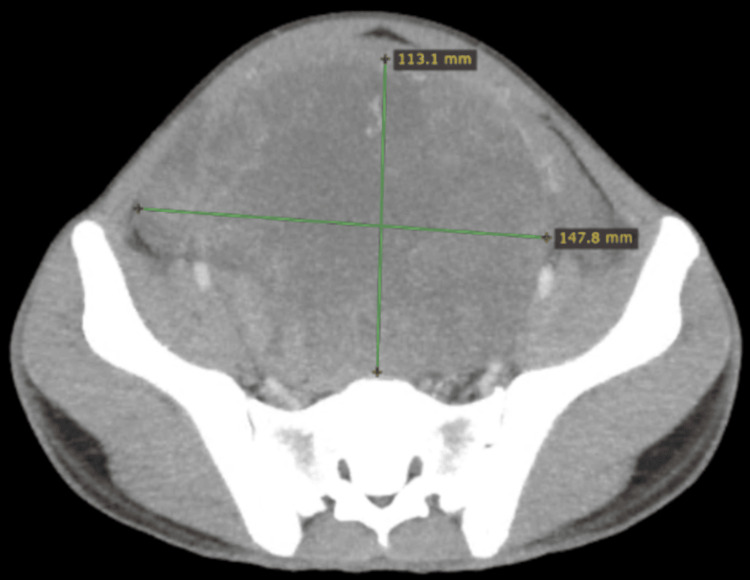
Axial planes of the CT scan showing a mass of around 11×15 cm CT: computed tomography
